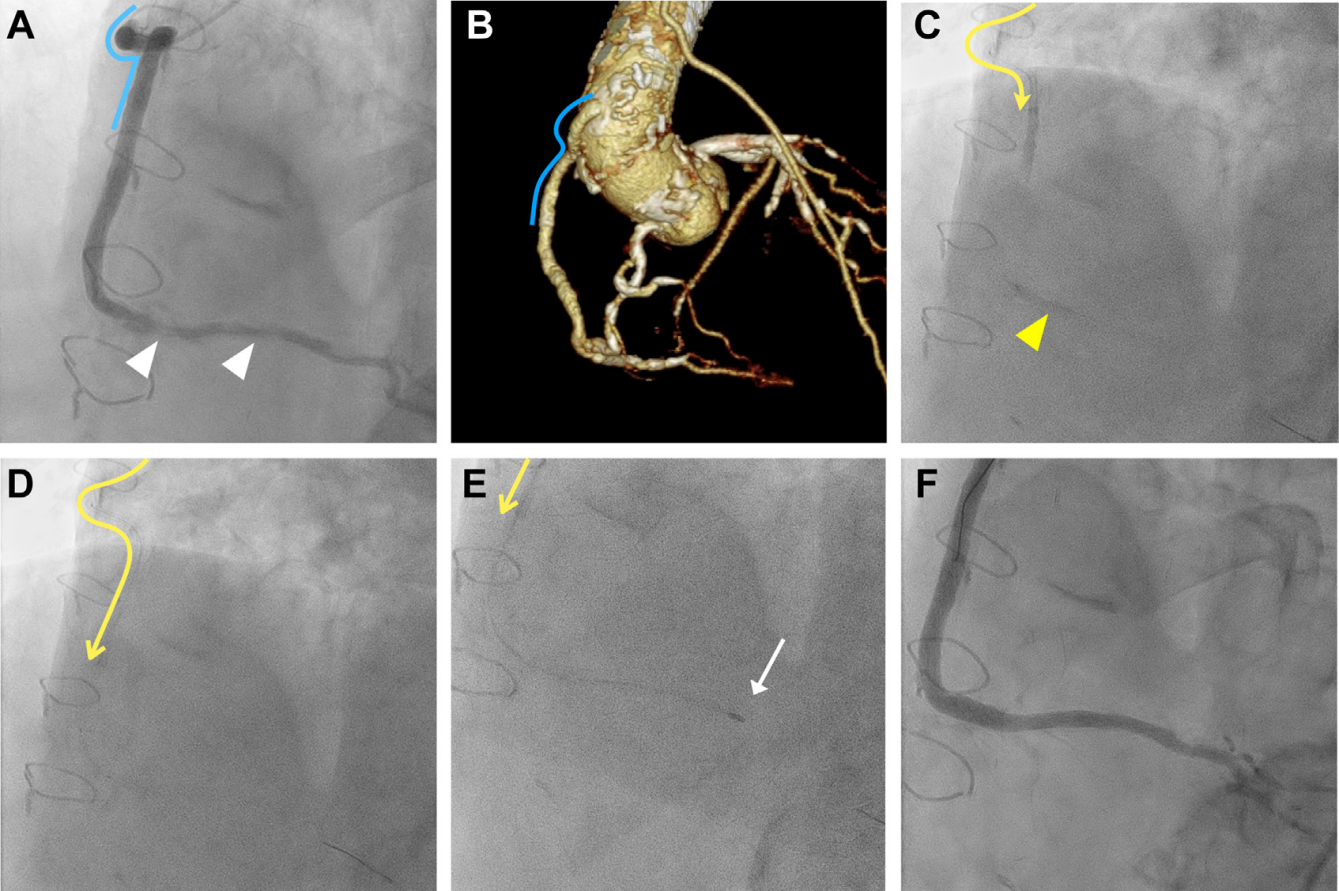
Deep Engagement of a 6-F Guide Catheter Through a Tortuous Saphenous Vein Graft and Rotational Atherectomy for Distal RCA Lesion (A and B) Baseline coronary angiography and computed tomography reveals calcified distal RCA stenosis and a significantly tortuous proximal saphenous vein graft (blue line) anastomosed to the mid-RCA. (C and D) A 6-F Judkins right 4.0 guide catheter (yellow line with arrow) is deeply inserted beyond the tortuous saphenous vein graft segment via distal balloon anchoring technique (yellow arrowhead). (E) Deeply inserted guide catheter enables safe delivery of the 1.5-mm burr (white arrow) distally and subsequent rotational atherectomy. (F) Final angiography demonstrates the excellent results. Abbreviation as in Figure 1.
